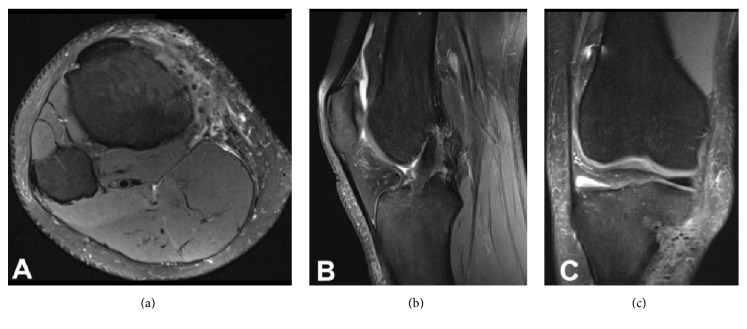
(a) T2 axial slice of the MRI demonstrating resolution of the pretibial fluid collection. (b) T2 sagittal image demonstrates an intact ACL graft resolution of tibial bone marrow edema. (c) T2 coronal image again demonstrates resolution of pretibial fluid collection and proximal tibial edema.
